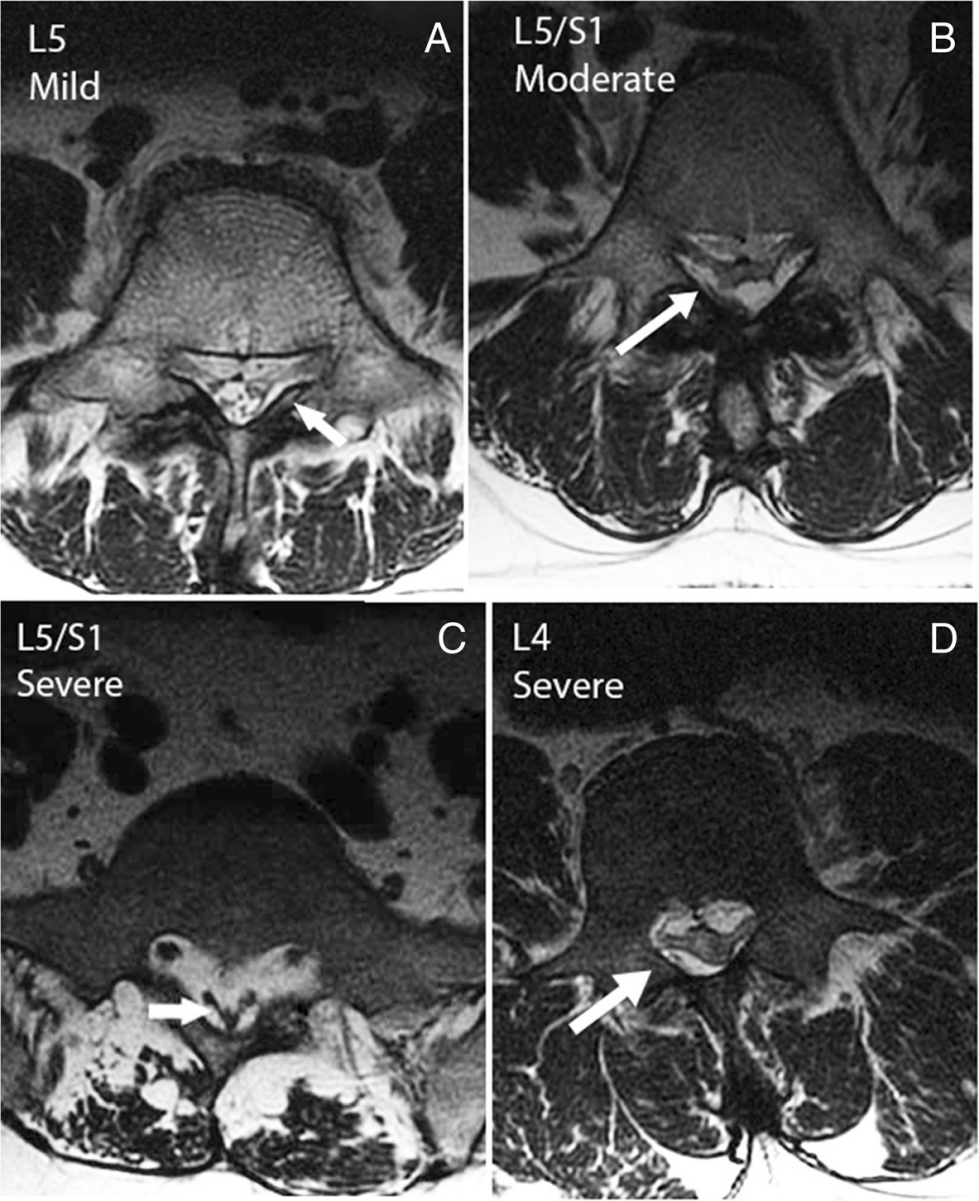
Severity of EL can be determined from physical features. (A) Axial image through the body of L5 with early EL. The L5 nerve roots are visible from thecal sac to Lateral Recesses. Epidural Lipomatosis (EL) enhances the visibility of the L5 roots. The arrow points to the indentation of the thecal sac. (B) Epidural fat tethering dural sac producing stellate appearance and moderate EL. (C) Axial MR image through the body of S1 with severe EL. The arrow points to the thecal sac in the shape of a Y (pathognomonic for severe EL). The buttock muscles are infiltrated with fat and the overlying subcutaneous tissue is excessive. (D) Axial MR image through the body of L4 with EL, compressing the roots and thecal sac. Since the neural elements normally occupy a larger area of the spinal canal at L4 than L5/S1, the compression by EL is considered severe.
